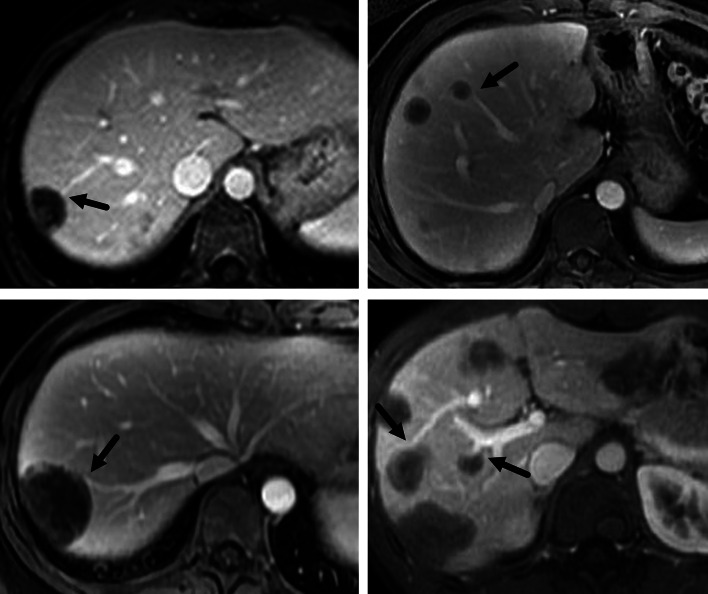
The appearance of lollipop sign on MRI images of 4 HEH patients (arrows)
